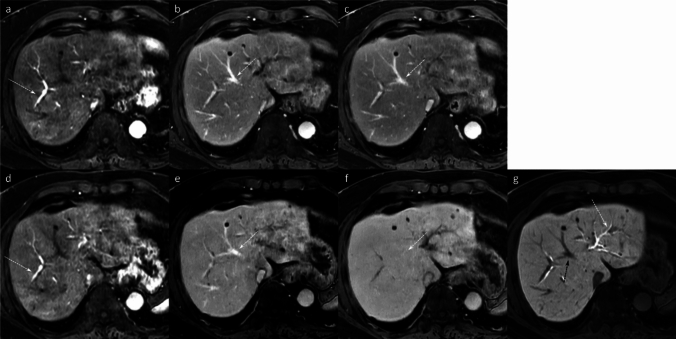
Comparison of the dynamic contrast-enhanced MRI using two different contrast agents. A 73-year-old man with pancreatic adenocarcinoma (not shown) underwent two MRI examinations within 1 week, each utilizing a different contrast agent. The first MRI was performed using an extracellular contrast agent (ECA) (a–c), and the second using gadoxetic acid (d–g). In the late hepatic arterial phase, both ECA (a) and gadoxetic acid (d) demonstrate marked arterial enhancement and early portal vein opacification (arrows). Although gadoxetic acid uptake in the hepatic parenchyma may begin as early as the portal venous phase, the appearance of the portal venous phase with ECA (b) and gadoxetic acid (e) is similar, with the middle hepatic veins (arrows) being hyperintense relative to the background parenchyma. At approximately 3 min post-injection, the delayed phase with ECA (c) and transitional phase with gadoxetic acid (f) are acquired. During this phase, gadoxetic acid distributes into both the extracellular and intracellular spaces, rendering the middle hepatic vein (arrow) isointense to the background parenchyma. In contrast, the middle hepatic vein (arrow) remains hyperintense to the background parenchyma on the delayed phase with ECA (c). In the hepatobiliary phase (g), gadoxetic acid is predominantly cleared from the extracellular space and accumulates mainly in the intracellular space and bile ducts. Consequently, the liver parenchyma appears more enhanced than the intrahepatic vessels (black arrow), and gadoxetic acid is visualized within the bile ducts (white arrow)
